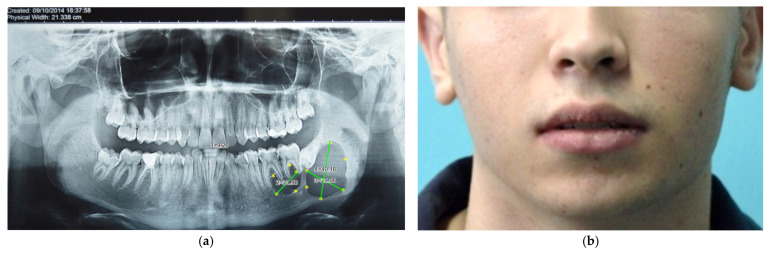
Initial orthopantomogram, with delineation of the bone lesion boundaries and corresponding image measurements (a), Exofacial aspect of the patient (b).
